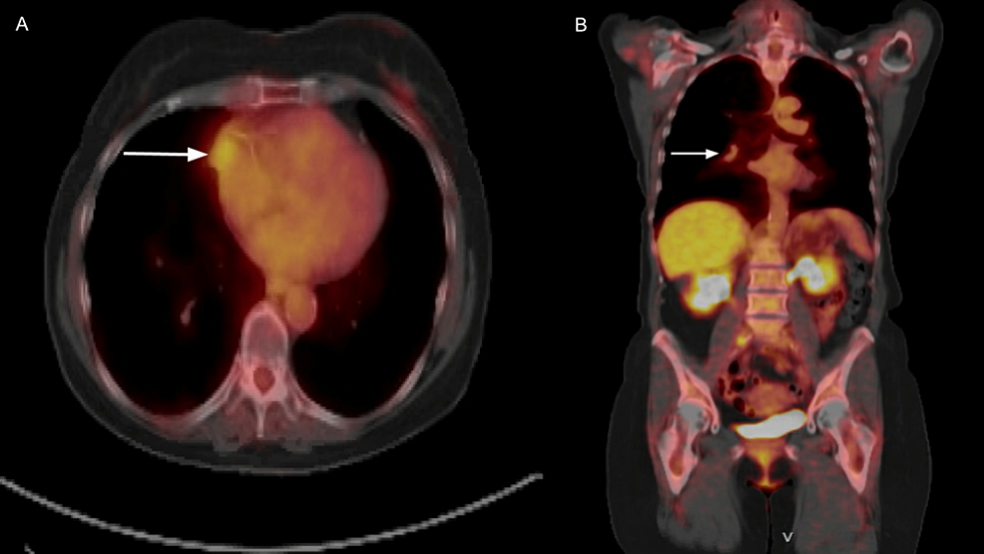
Follow-up PET-CT revealed mild activity (white arrows) in the right middle lobe (A: axial view, B: coronal view). Although the area appeared slightly larger relative to the prior, it showed low-grade activity. PET-CT: Positron emission tomography-computed tomography.
